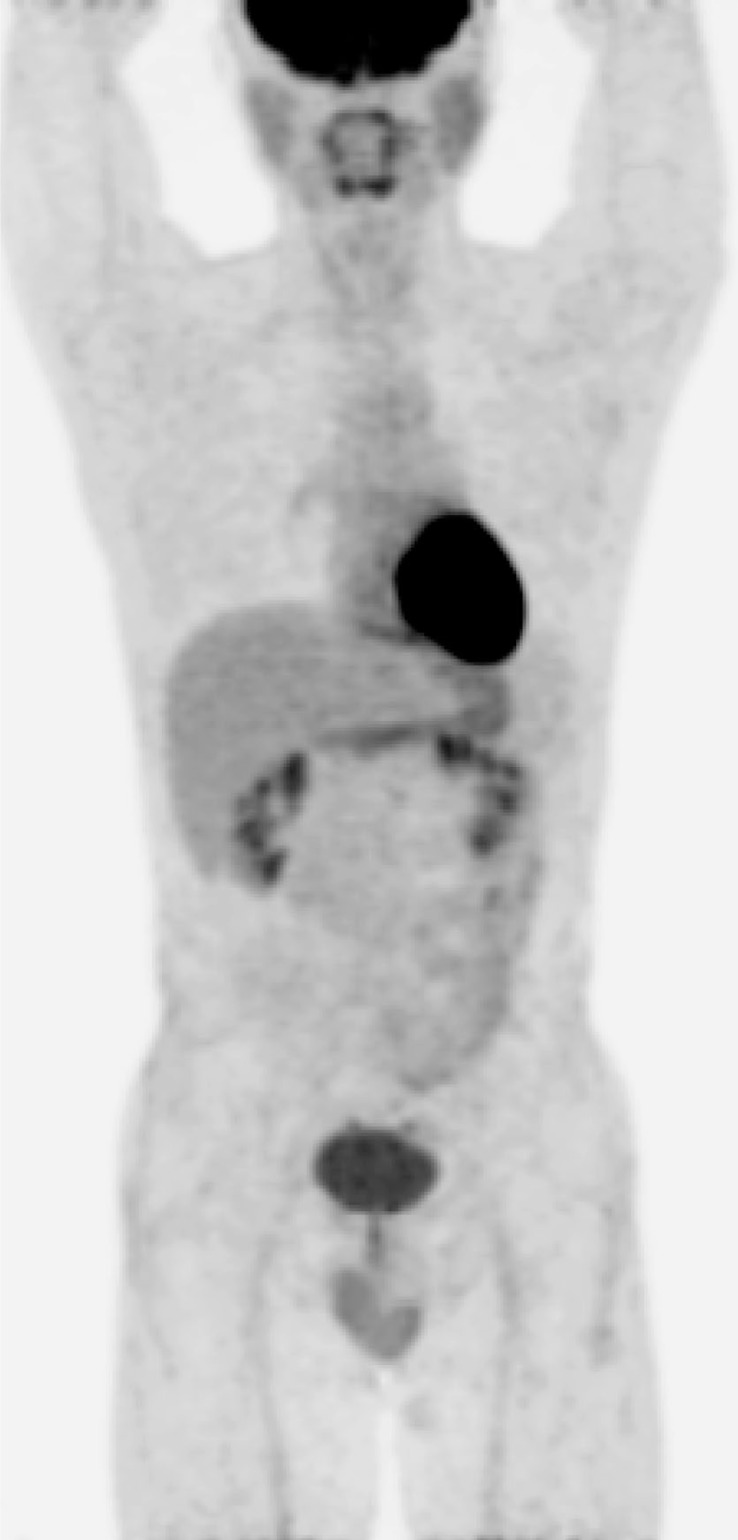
After three cycles of chemotherapy, the MIP image of follow-up F-18 FDG PET/CT showed complete metabolic remission of the involved lesions.
